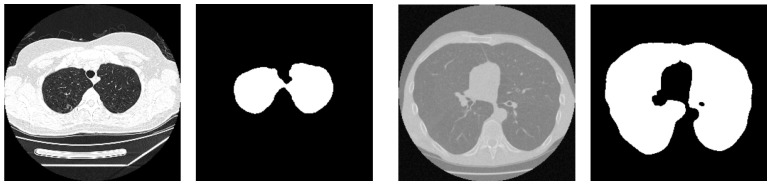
Visual comparison of preprocessing steps: original CT image, refined mask after preprocessing.
